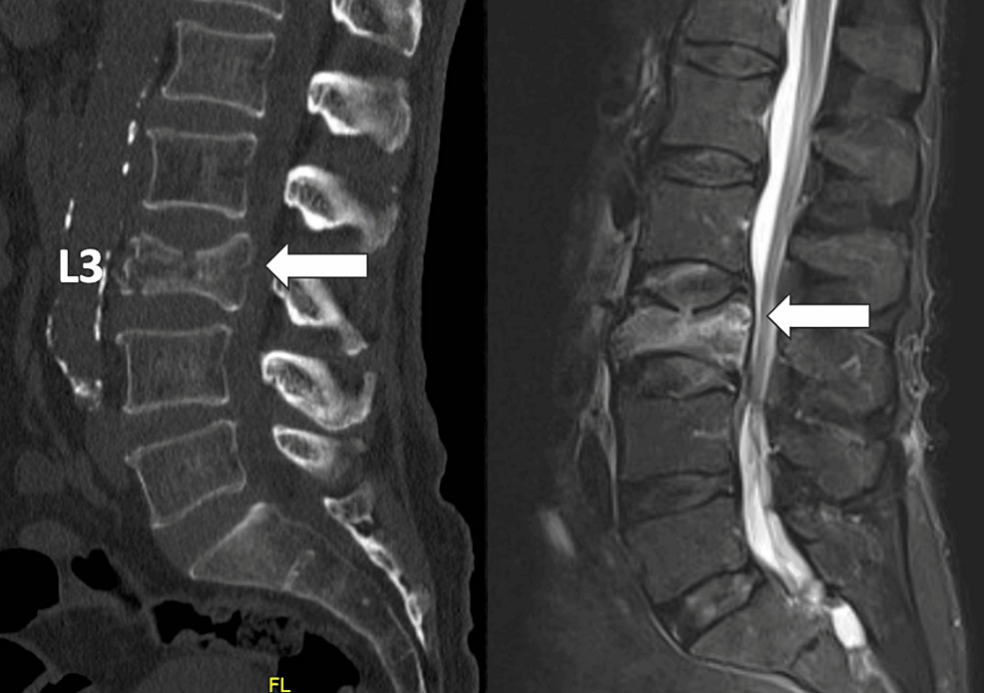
Bone window CT scan (A) and MRI STIR sequence (B) of the thoracolumbar spine. L3 with fracture of the posterior wall
